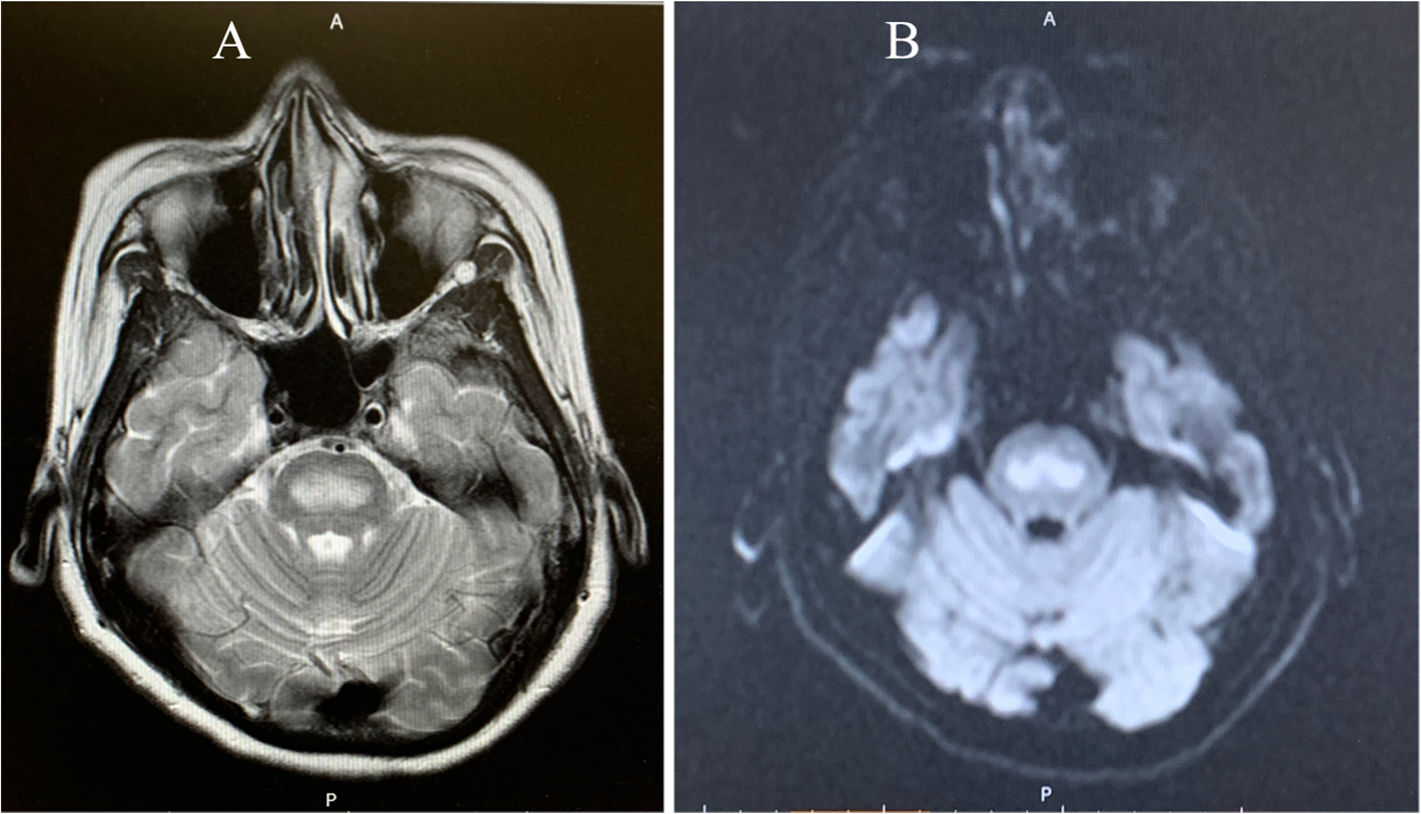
Cerebral magnetic resonance imaging showing hypersignals in the central pons and medial cerebellum in both the T2 weighted (a) and the diffusion-weighted images (b)
